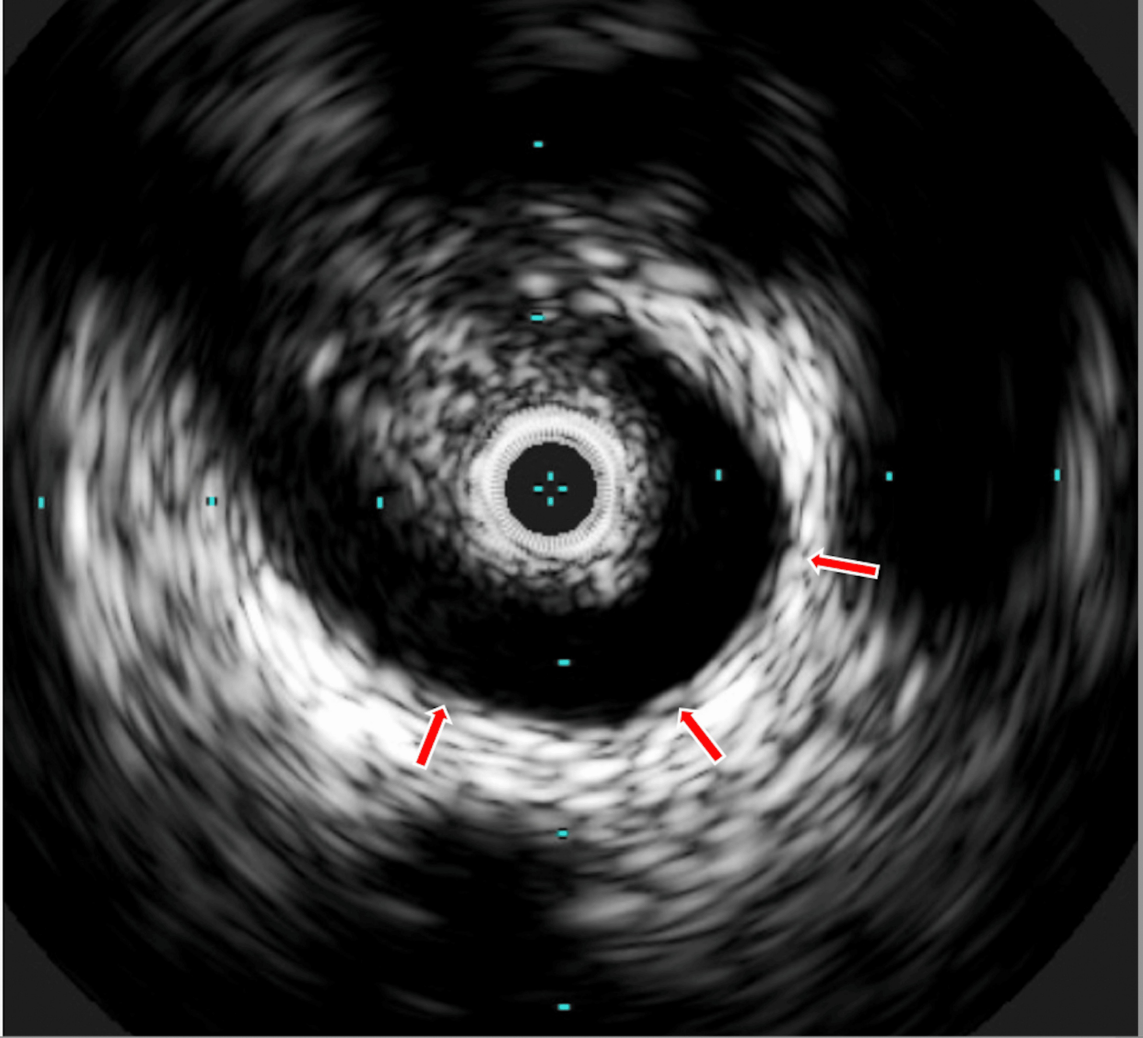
Intravascular ultrasound from inside the right hepatic duct at the level of the narrowing shows the immediately adjacent right hepatic artery (red arrow).
